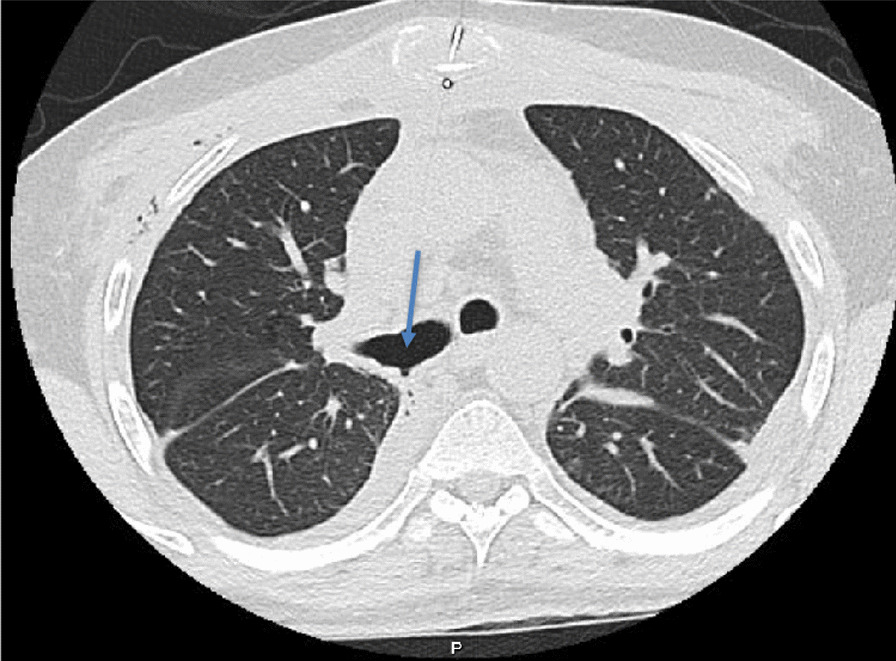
Day 35 post-transplant chest CT showing partial dehiscence and a small bulging contour along the posterior aspect of the right bronchial anastomosis with adjacent tiny air foci (arrow) concerning a partial dehiscence
